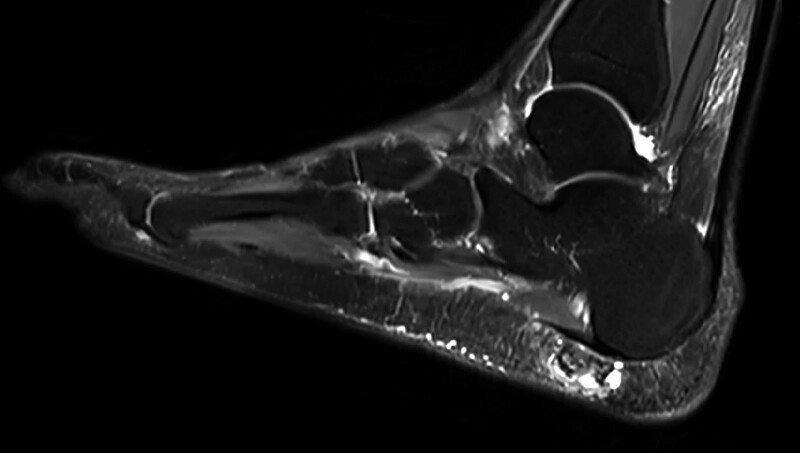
MRI scan of the right foot showing an abnormal signal shadow in the superficial fascial soft tissue of the right sole. MRI = magnetic resonance imaging.
